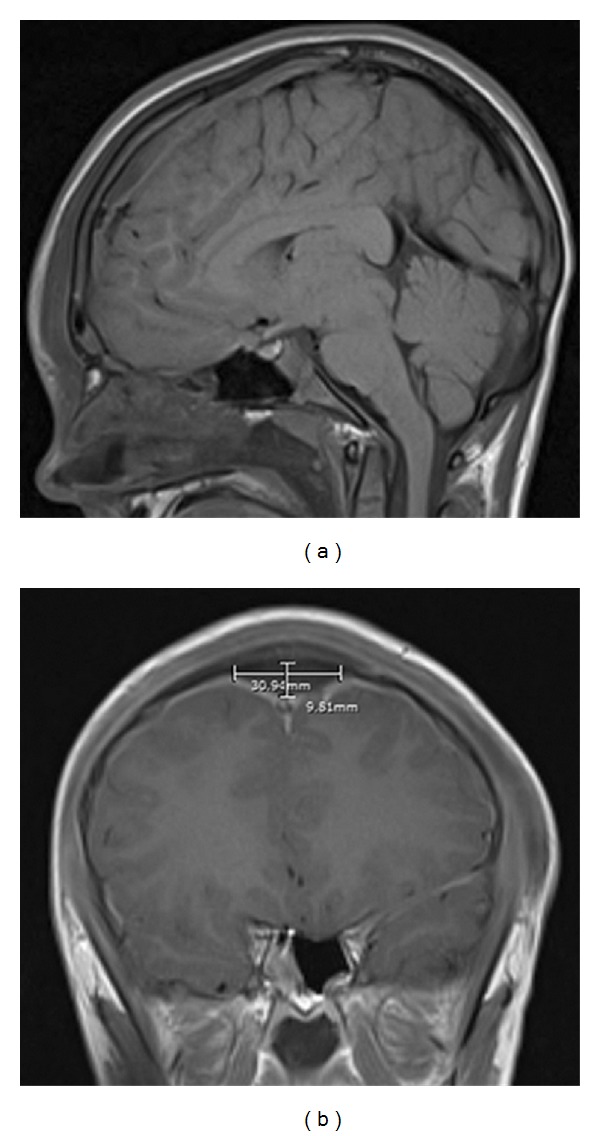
(a) MRI of the brain showing sagittal T1-weighted image after administration of contrast. The fluid collection reported to have a thin enhancing wall ran along the dorsal aspect of the superior sagittal sinus. (b) MRI of the brain showing coronal T1-weighted image after administration of contrast. Diffuse smooth dural enhancement is noted, bilaterally.
